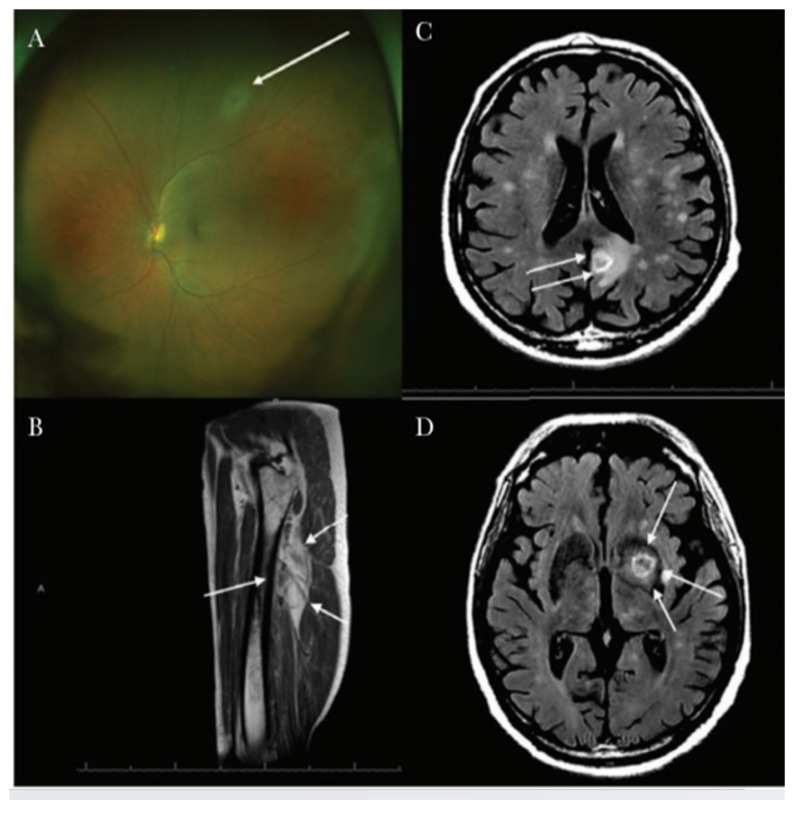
Case of disseminated toxoplasmosis on trametinib after acute *Toxoplasma* infection [5]: A 65-year-old male with rheumatoid arthritis and right orbit pseudolymphoma, required the addition of trametinib due to disease progression despite multimodal therapy. Five weeks later, he developed gait incoordination and fine motor skills deficits. The patient had CSF lymphocytic pleocytosis, retinitis ((A) shows left eye fundus with retinal whitening in the superior area consistent with retinitis [white arrow] without overlying vitritis on exam), myocarditis and myositis ((B) shows T1 MRI image of the right thigh consistent with myositis, with increased fluid signal in vastus intermedialis muscle [white arrows]). Brain MRI (T2 FLAIR [fluid-attenuated inversion recovery] images) showed post contrast, multiple ring enhancing lesions of toxoplasmosis [white arrows] (C,D). Serology suggested acute *Toxoplasma* infection. The patient reported recent consumption of wild boar sausages while on trametinib. After a 16-week course of high dose TMP-SMX, the patient had substantial clinical improvement; at 4 months follow up, there was a reduction in size of the CNS lesions. (Reproduced with permission from Gharamti AA et al. Acute *Toxoplasma* Dissemination with Encephalitis in the Era of Biological Therapies. *Open Forum Infectious Diseases*, Nov 2018; 5(11):ofy259, doi:10.1093/ofid/ofy259) [5].
